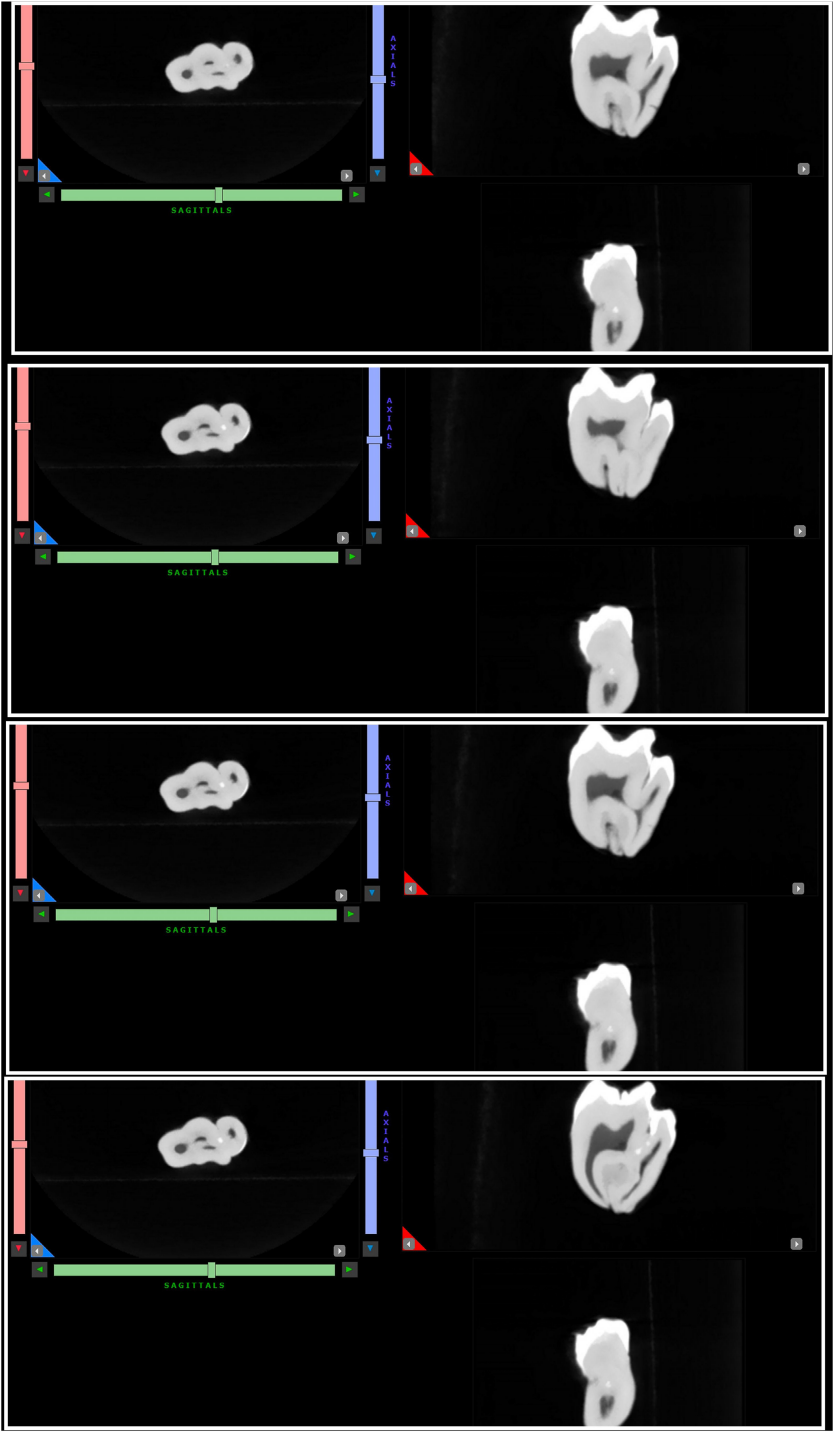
Images exported from ex vivo CBCT of extracted double tooth. Shared pulp canal system is noticed.
